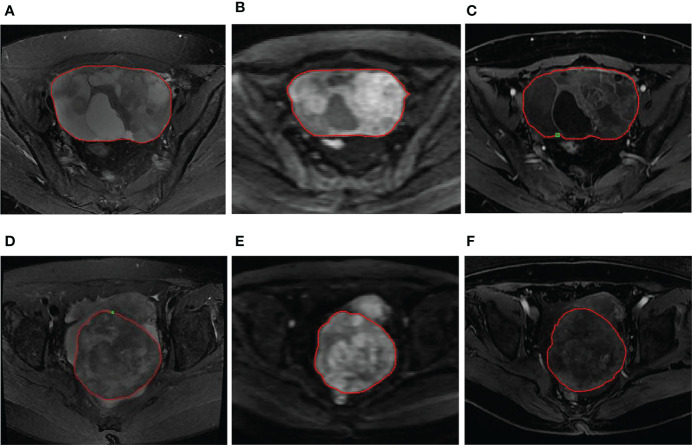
(A–C) A 56-year-old woman with LGSOC in the right ovary. (A) Axial FS-T2WI shows a mixed cystic-solid mass. (B) Axial DWI (b=1000 s/mm^2^) shows high signal of the solid component, indicating limited diffusion. (C) Axial T1WI+C image shows a mild enhancement in the solid component and septation. The red ROI was manually drawn along the margin of the whole tumor. (D–F) A 63-year-old woman with HGSOC in the right ovary. (D) Axial FS-T2WI shows a mass with mixed signals dominated by solid components. (E) Axial DWI (b=1000 s/mm^2^) shows high signal of the tumor, indicating limited diffusion. (F) Axial T1WI+C image shows a heterogeneous mild enhancement in the tumor. The red ROI was manually drawn along the margin of the whole tumor.
